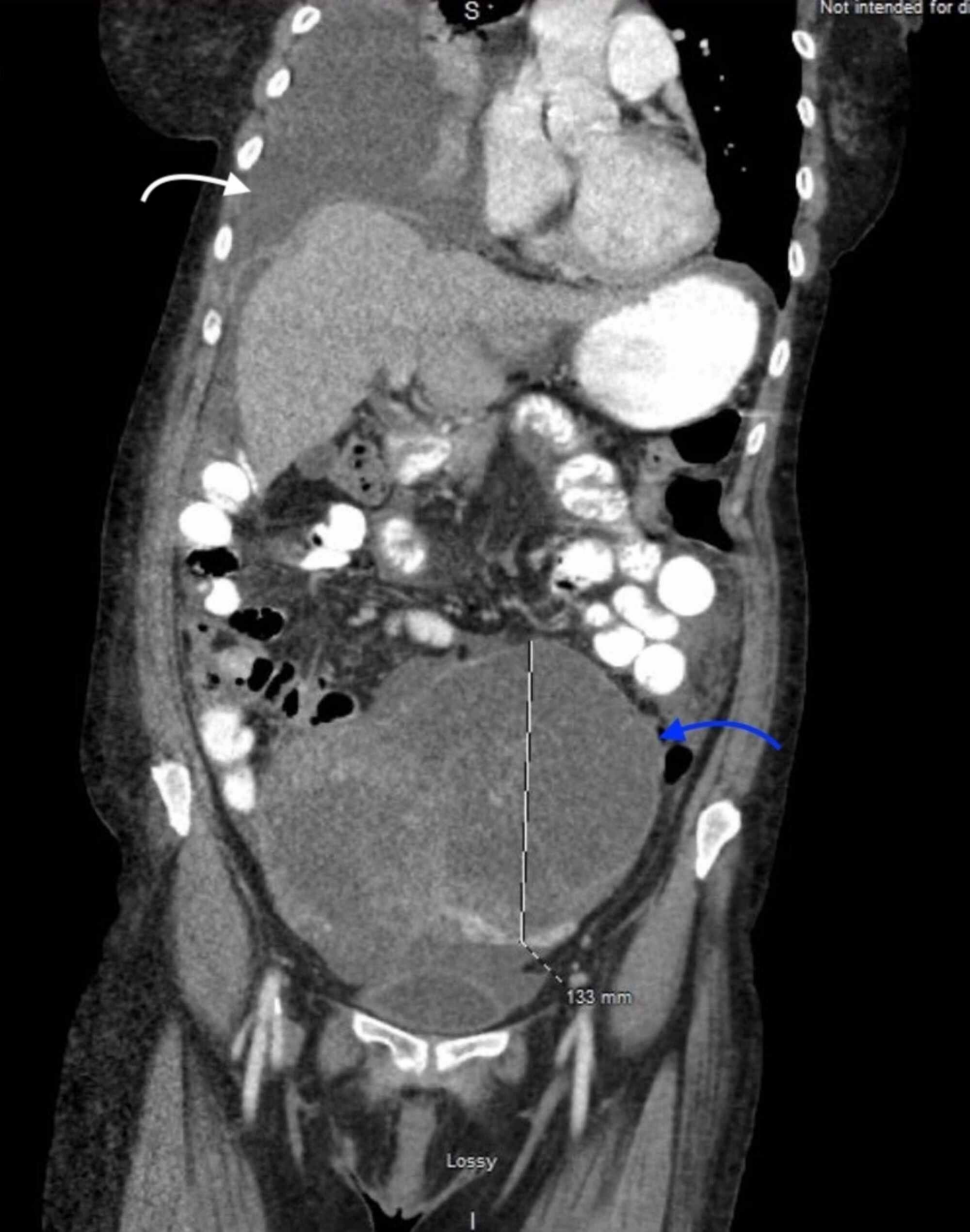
CT scan showing pleural effusion (white arrow) and a large pelvic mass (blue arrow).
